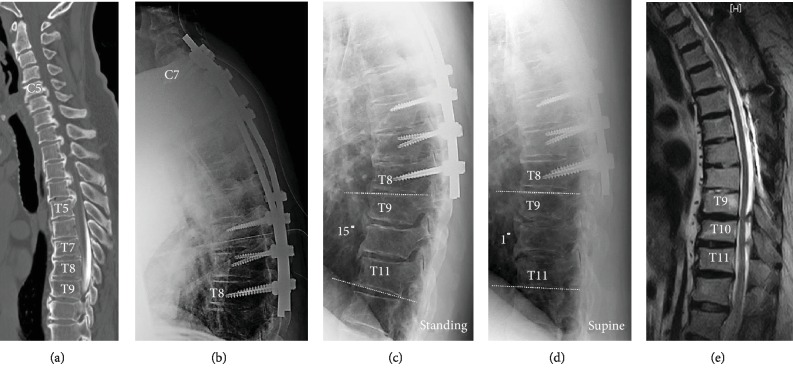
Spinal imaging before and after the initial surgery and before reoperation. (a) Ossification of the posterior longitudinal ligament was observed from C5 to T5. Bony fusion of the anterior longitudinal ligament was also observed at T7-9. (b) After initial surgery involving C3-6 laminoplasty and C7-T8 posterior decompression and fusion. (c, d) The kyphosis angle in T9-T11 was 15 degrees in the standing position (c) and 1 degree in the supine position (d) before reoperation. (e) MRI T2-weighted sagittal section. Spinal decompression in the initial surgical range was good. Advanced ossification of the yellow ligament at T9-11 and spinal cord compression at T9-10 are shown.
